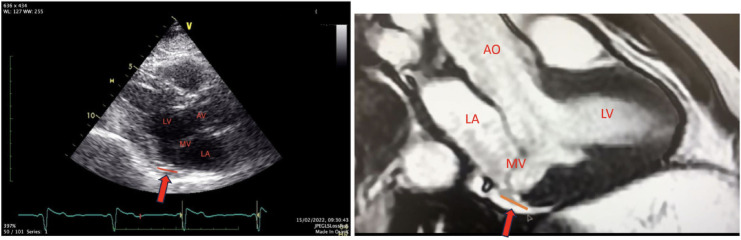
Transthoracic and MRI long-axis views. The red line and red arrow indicate the MAD area.
